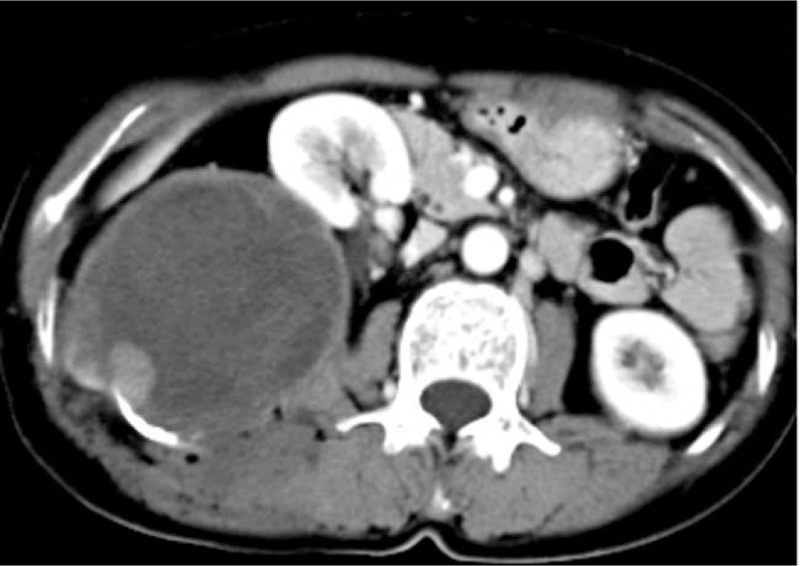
Solid components of CT-enhanced masses were unevenly enhanced and some cyst walls were nodularly enhanced, and the cystic lesion showed no enhancement.
